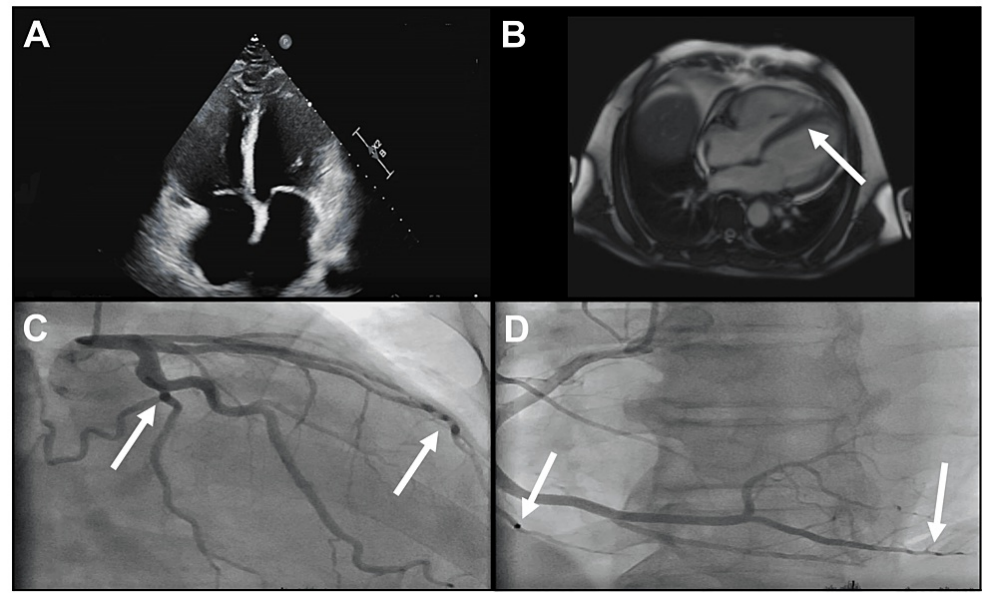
Cardiac imaging in a patient with Chagas cardiomyopathy (a) Transthoracic echocardiogram in four-chamber view showing dilation of all chambers. (b) Cardiac magnetic resonance imaging (MRI) showing myocardial fibrosis of the interventricular septum (c) and (d) coronary angiography showing non-occlusive anatomy in the arterial territory of the left main and its branches as well as the right coronary arteries.
